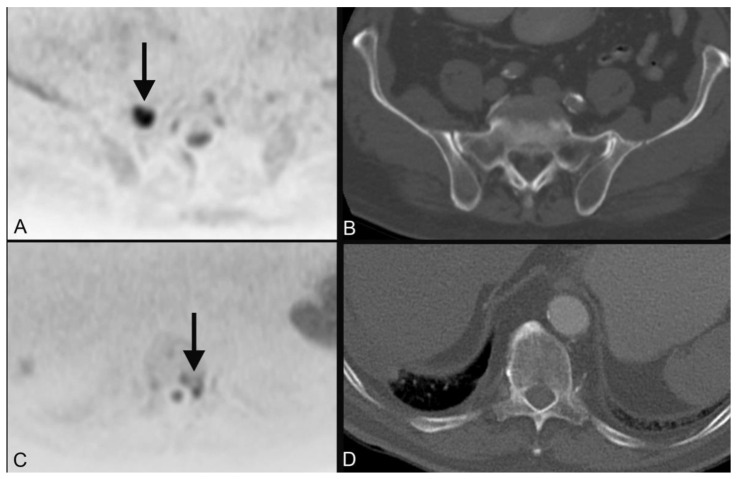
An 85-year-old male patient who was diagnosed with cancer of the right palatine tonsil (cT1 cN2b cM0) showed diffuse spotty diffusion restrictions in DWIBS of the skeletal system with the largest lesions in the right sacral mass (A, black arrow) and the 11th thoracic vertebrae on the left (C, black arrow) without correlate in the CT (B,D) and was later diagnosed with multiple myeloma.
